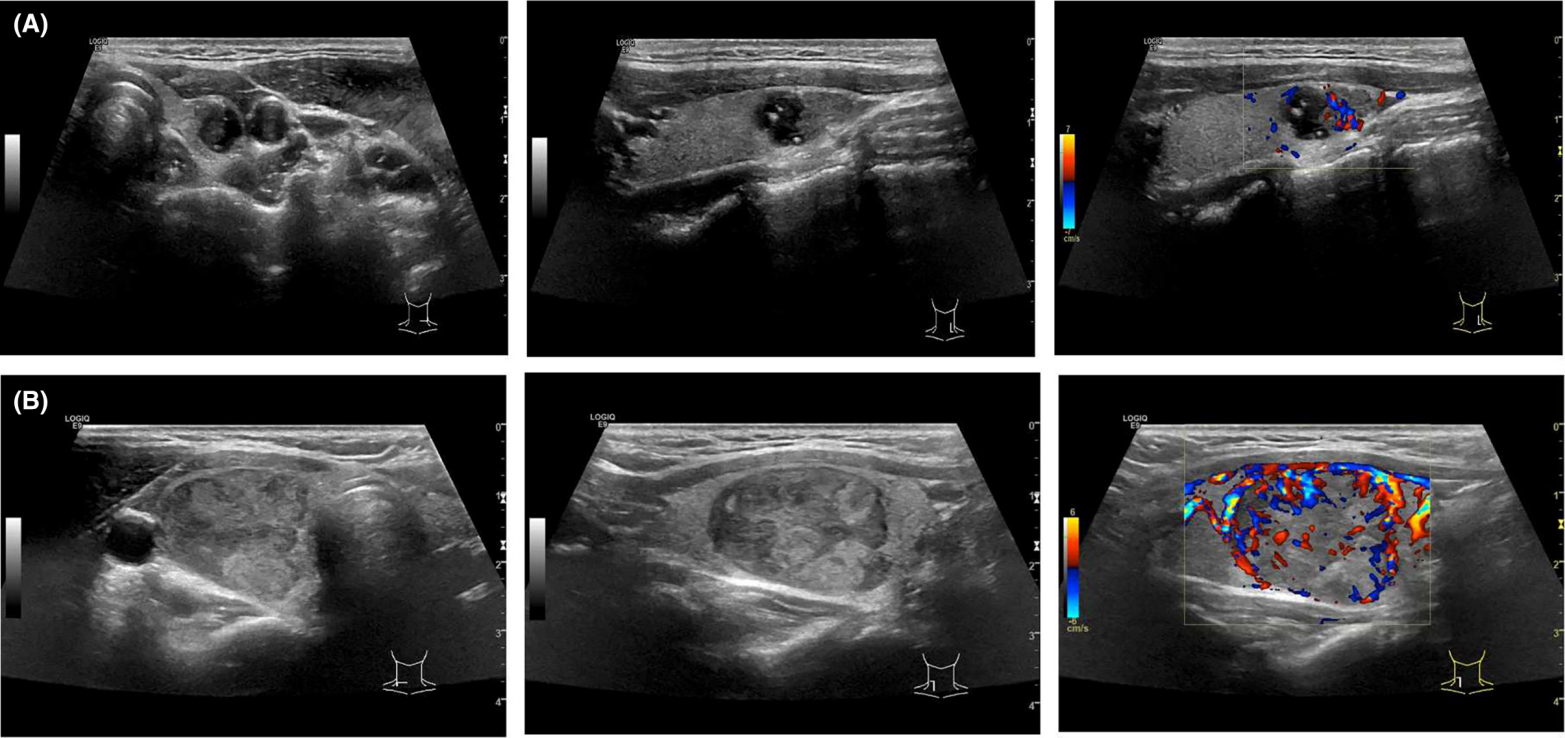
(A) Ultrasound images of PTC. (B) Ultrasound images of FTC.
